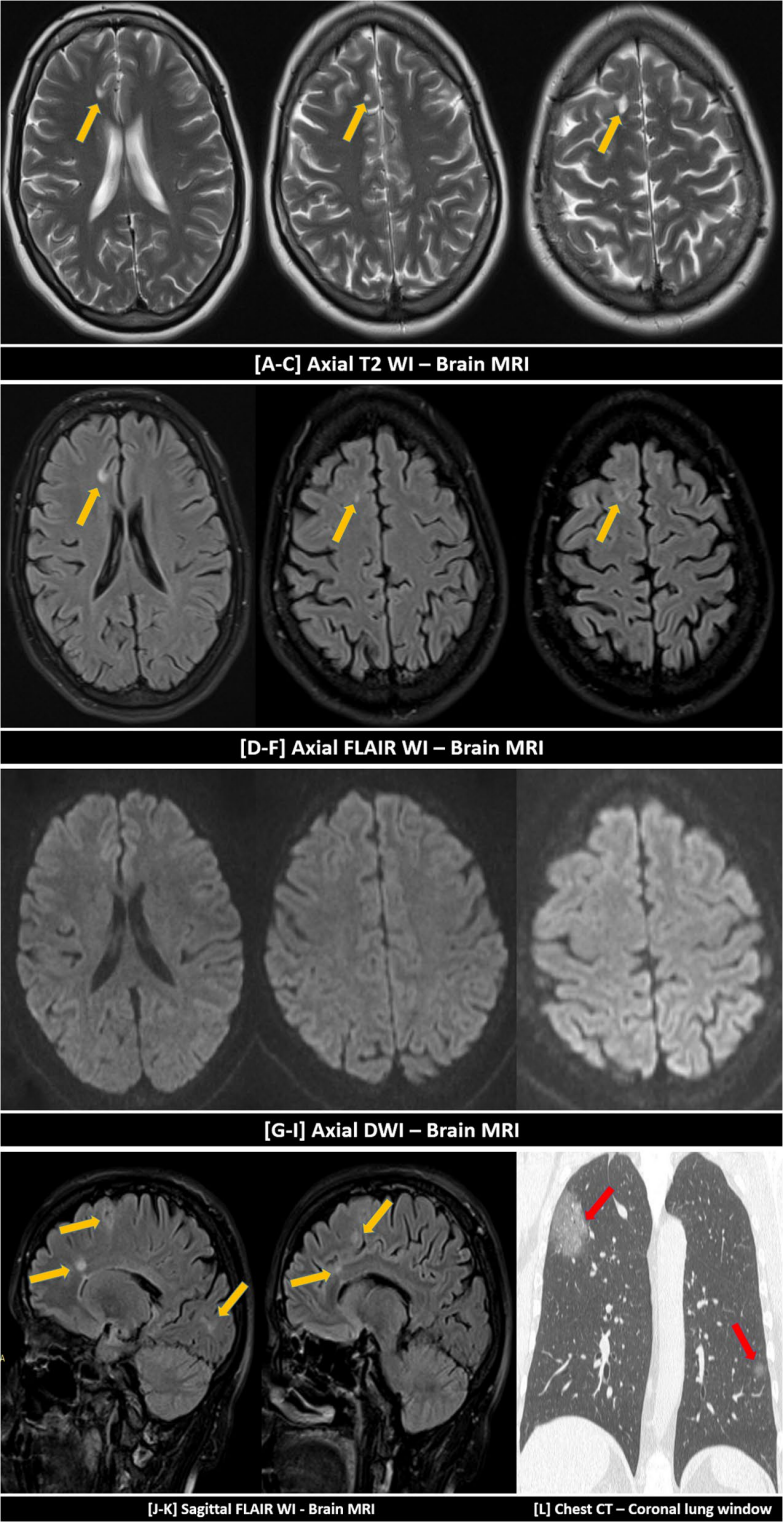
A 39-years-old female patient, with a history of COVID-19 infection two months ago, complained of dizziness. Partial improvement was achieved on supportive treatment. MRI was requested because of persistent symptoms. No previous history of neurological diseases. The neurological examination was free. Multiple small cortical and to a lesser extent sub-cortical as well as deep white matter lesions are seen in the right frontoparietal lobes (yellow arrows) showing: T2 hyper-intense signal [A–C], FLAIR hyper-intense signal [D–F] without DWI restriction [G–I]. [J, K] Sagittal FLAIR weighted images are demonstrating the lesions. [L] Coronal chest CT (lung window) 2 months ago showed a right apical sub-pleural ground-glass patch of COVID-19 infection. Symptoms alleviated after a short course of steroid therapy with resolution of MRI lesions… both, the clinical context and cortical involvement, suggested the possibility of post-COVID vasculitis rather than demyelinating diseases
